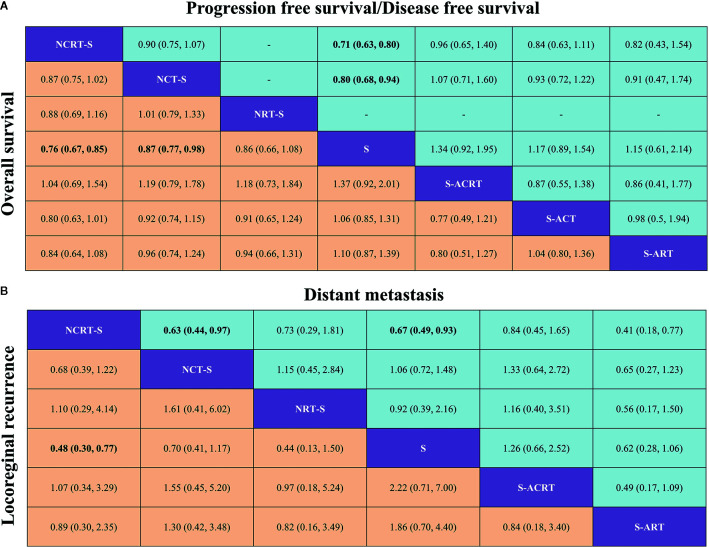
(A) Pooled relative effects of all treatment comparisons in the NMA. Pooled HRs (95% CrIs) for PFS/DFS (upper triangle) and OS (lower triangle). Data in each cell are HRs (95% CrIs) for the comparison of row- versus column-defining treatment for PFS/DFS or the comparison of column- versus row-defining treatment for OS. Statistically confident results are in bold. NCRT-S, neoadjuvant chemoradiotherapy followed by surgery; NCT-S, neoadjuvant CT followed by surgery; NRT-S, neoadjuvant RT followed by surgery; S, surgery; S-ACRT, surgery followed by adjuvant CRT; S-ACT, surgery followed by adjuvant CT; S-ART, surgery followed by adjuvant RT. (B) Pooled relative effects of all treatment comparisons in the NMA. Pooled ORs (95% CrIs) for distant metastasis (upper triangle) and locoregional recurrence (lower triangle). Data in each cell are ORs (95% CrIs) for the comparison of row- versus column-defining treatment for distant metastasis or the comparison of column- versus row-defining treatment for locoregional recurrence. Statistically confident results are in bold. NCRT-S, neoadjuvant CRT followed by surgery; NCT-S, neoadjuvant CT followed by surgery; NRT-S, neoadjuvant RT followed by surgery; S, surgery; S-ACRT, surgery followed by adjuvant CRT; S-ACT, surgery followed by adjuvant CCT; S-ART, surgery followed by adjuvant RT.
